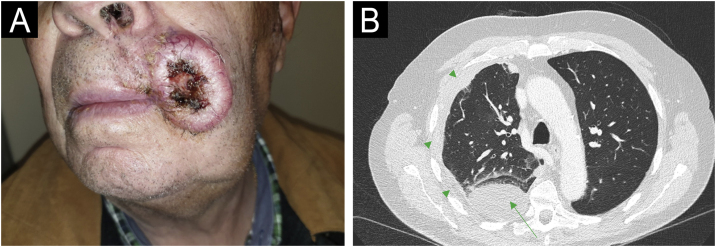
Patient Number 2. For Patient 2, a cSCC measuring 4.2 × 3.5 cm is observed at the left commissure of the lips (A). The axial CT scan (B) displays a mass consistent with pulmonary metastasis in the posterior segment of the lower right lobe (green arrows), accompanied by pleural effusion (green arrowheads) and associated laminar atelectasis.
